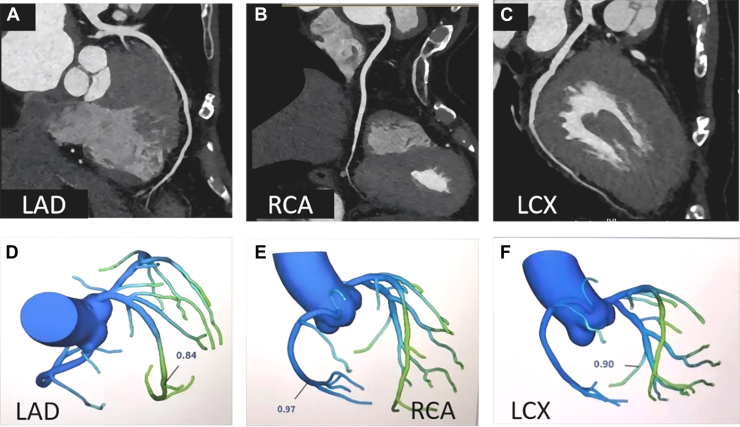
Coronary Computed Tomography Angiography and CT-Derived Fractional Flow Reserve Findings (A to C) Coronary computed tomography angiography images: (A) A myocardial bridge in the mid segment of the left anterior descending artery (LAD). (B and C) No significant stenosis in the left circumflex (LCX) or right coronary artery (RCA). (D to F) CT-derived fractional flow reserve analysis: The values were 0.84 in the LAD (D), 0.90 in the LCX (E), and 0.97 in the RCA (F), indicating no functionally significant ischemia.
